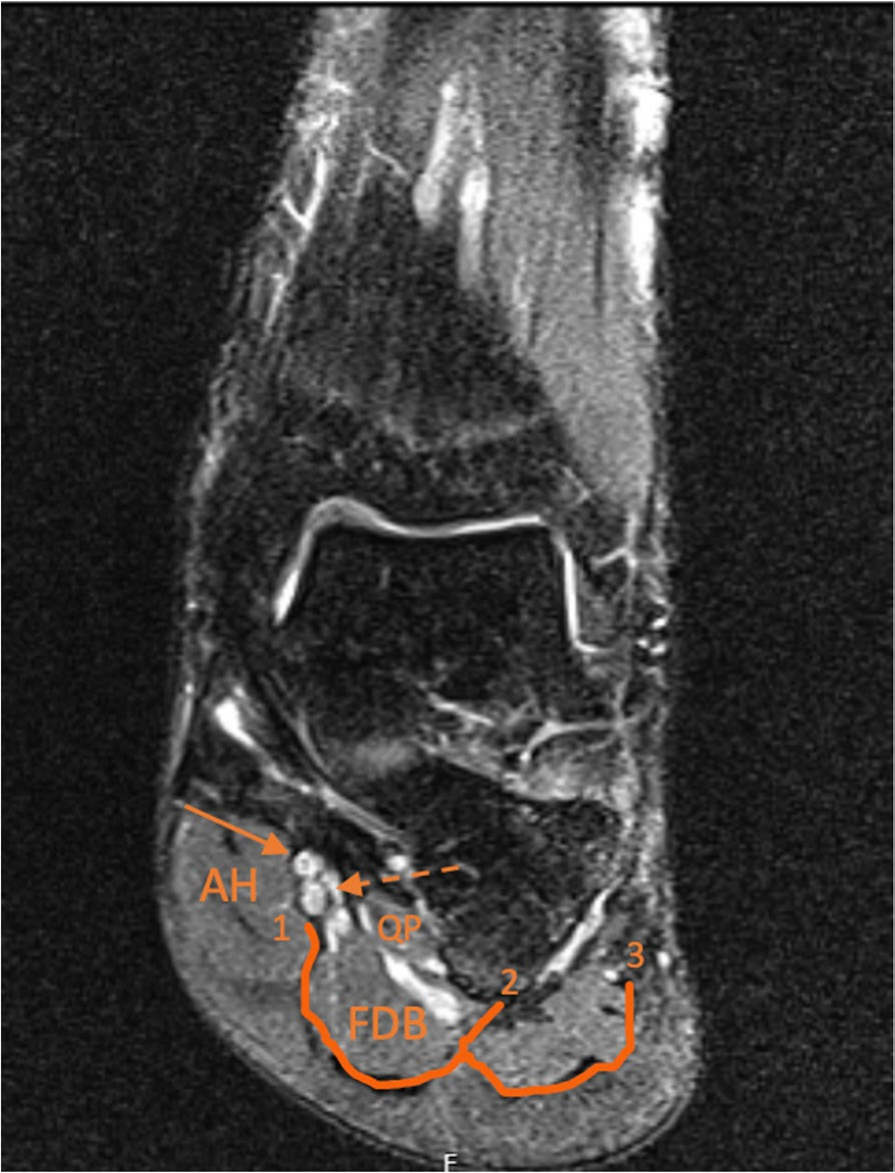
Coronal T2 weighted magnetic resonance image of a 39 year old male left ankle and foot. 1: Medial Septum; 2: Intermediate septum; 3: Lateral Septum. AH: Abdutor Hallucis; FDB: Flexor Digitorum Brevis; QP: Quadratus Plantae; Dashed Arrow: lateral plantar nerve (LPN). Non-dashed arrow: medial plantar nerve (MPN)
